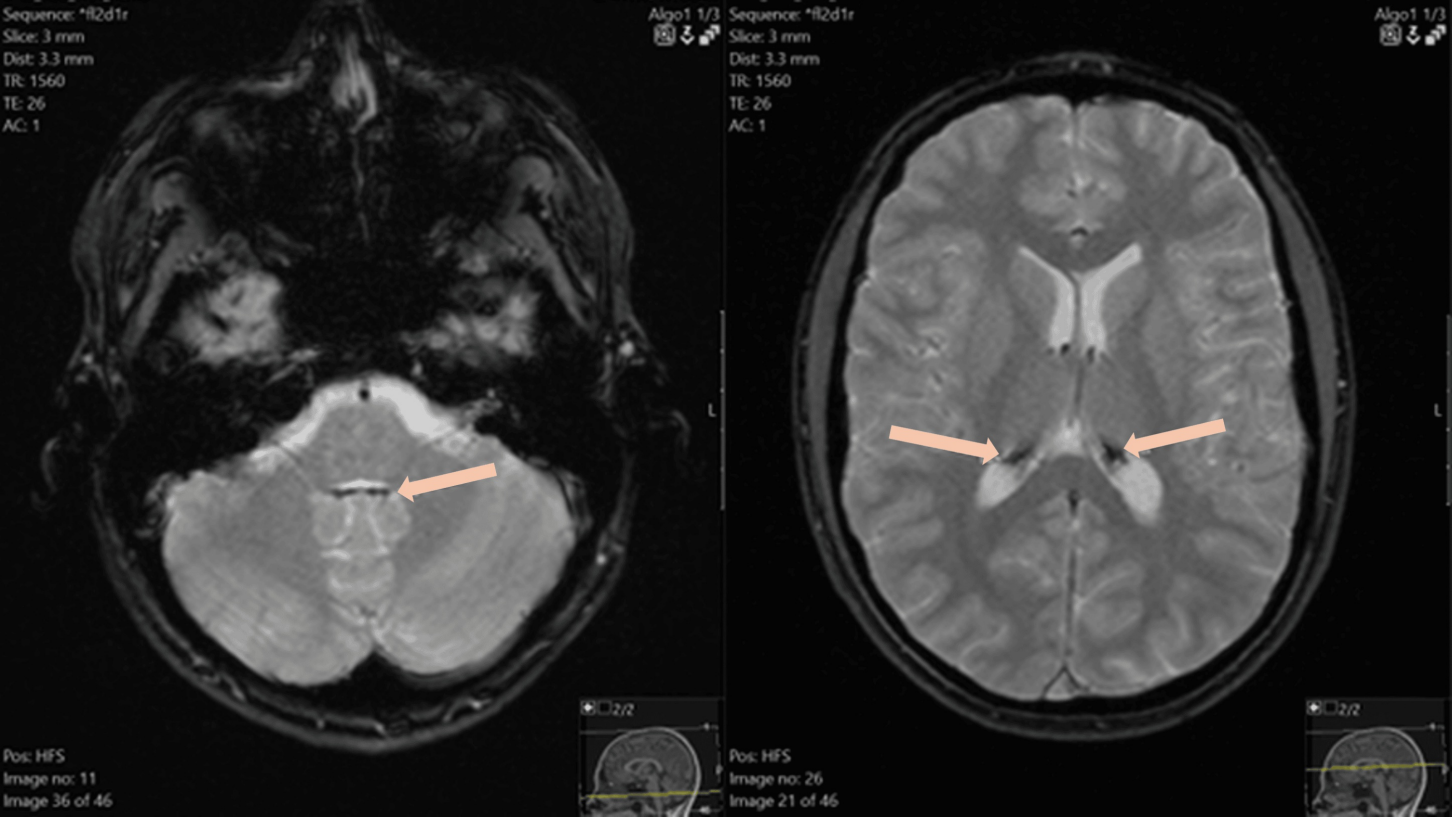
Brain MRI of a 14-year-old male with HR B-ALL and secondary cerebral iron deposits Figure displays MRI findings indicative of brain IO - signal decline visible in the choroid plexuses of the lateral ventricles and the IV ventricle. ALL: acute lymphoblastic leukemia, HR: high-risk, IO: iron overload, MRI: magnetic resonance imaging
